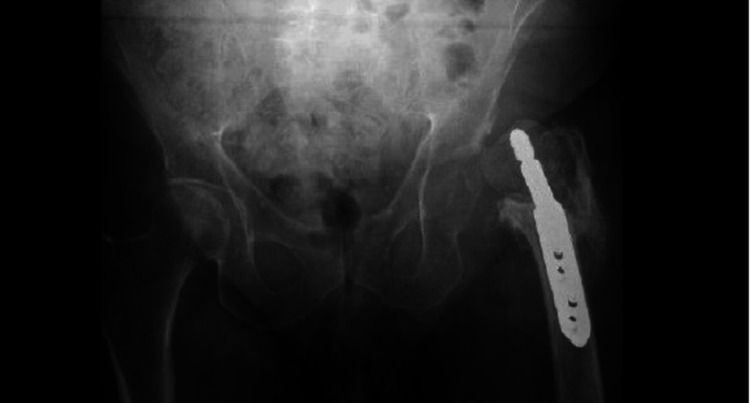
Pelvic X-ray anteroposterior view The image shows the dislocated left hip
